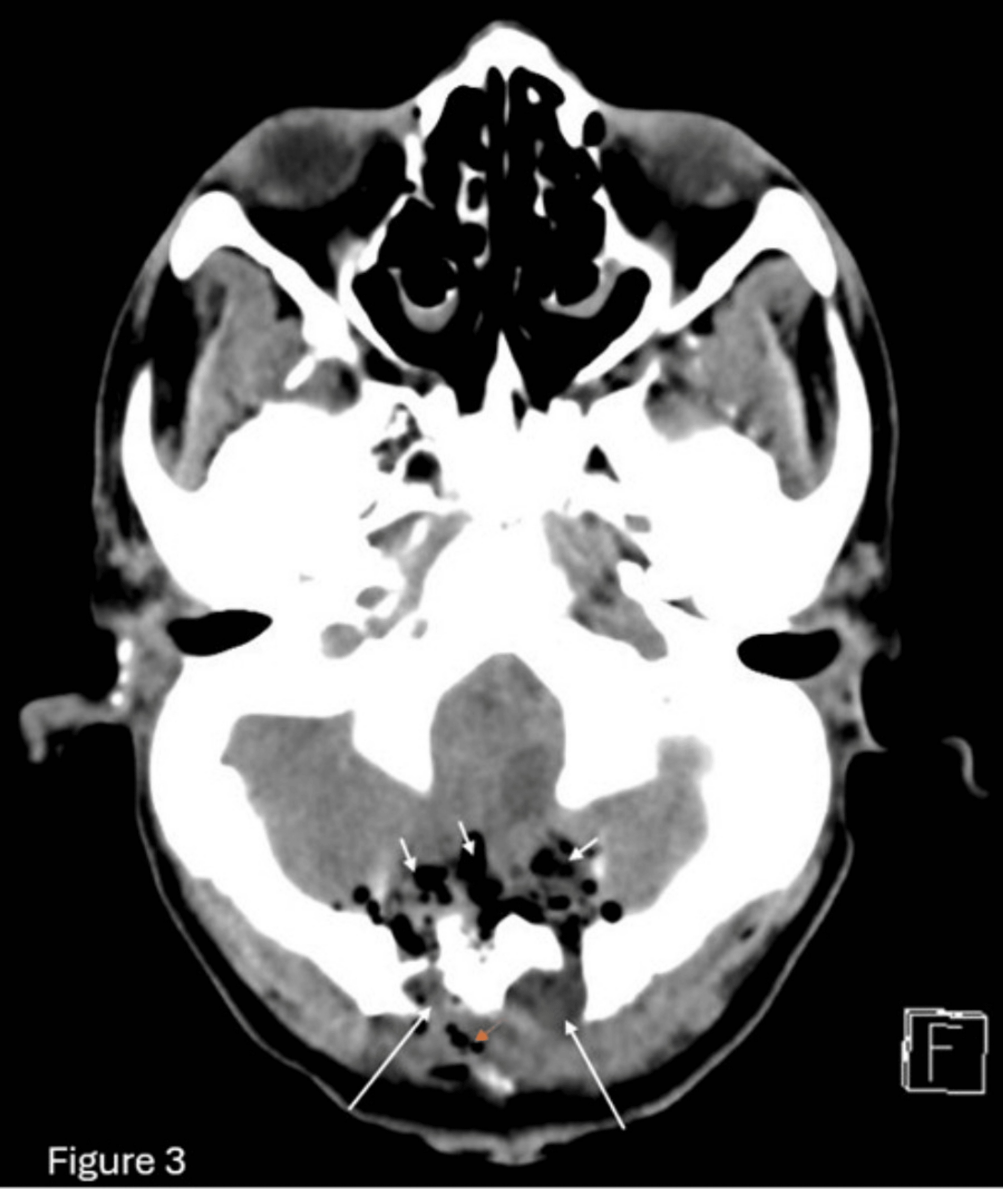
CT image showing postoperative posterior fossa craniotomy with pneumocephalus, surgical emphysema, and mild extracranial soft tissue swelling
